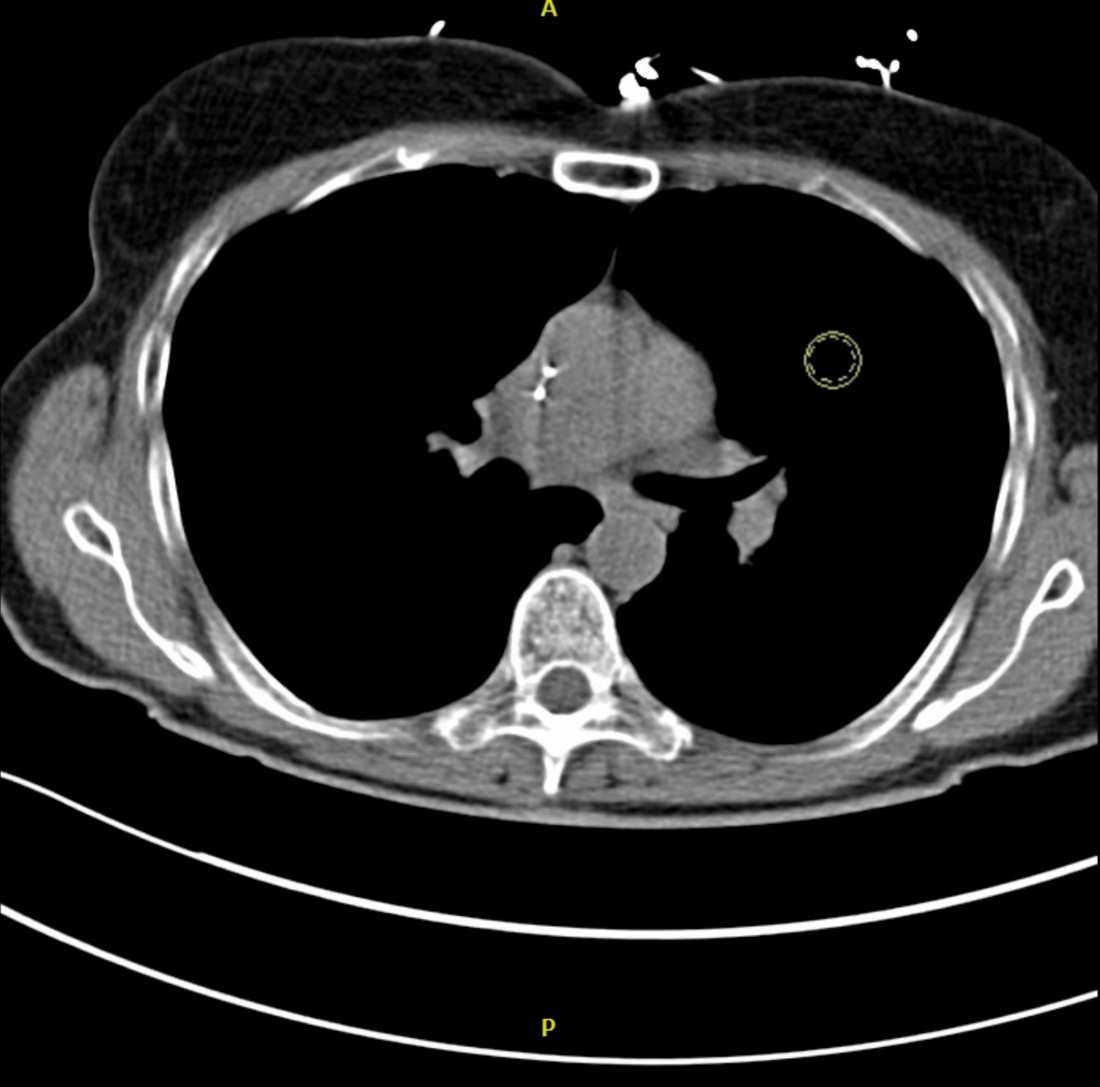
CTA of the chest showing no evidence of pulmonary embolism CTA: computed tomography angiography
